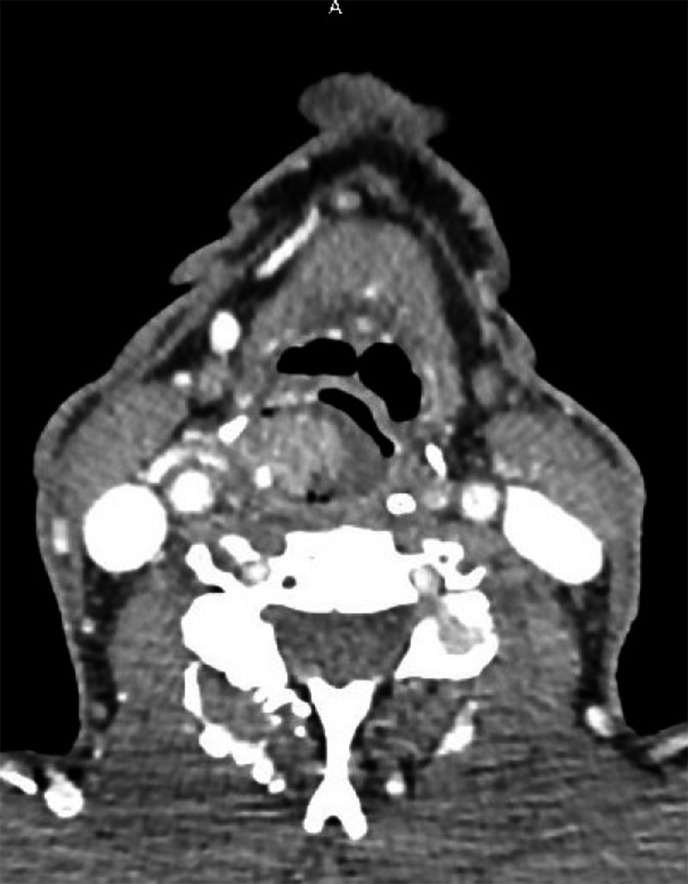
CT scan of the neck with contrast medium showing an oval mass of 25 mm of the right pyriform sinus
